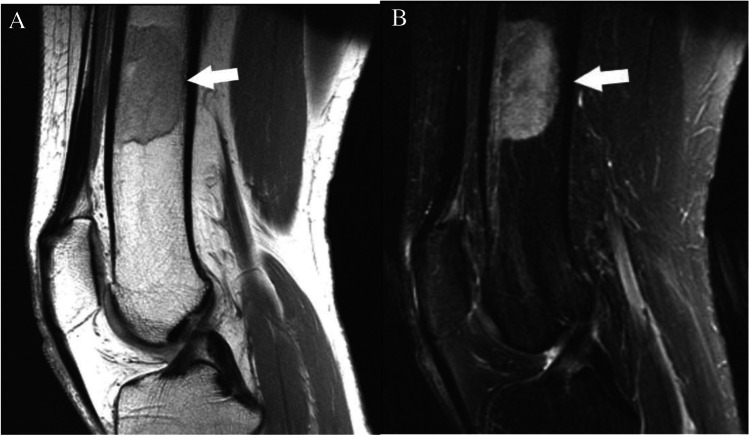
Case 8. Red marrow. 39-year-old female presents with knee pain suspicious for meniscal tear. There is an incidentally discovered lesion (arrows) in the distal femoral shaft that is slightly hyperintense to skeletal muscle on the A sagittal T1-weighted MR image and hyperintense on the B sagittal T2 fat-suppressed MR image. This finding is compatible with focal red marrow
